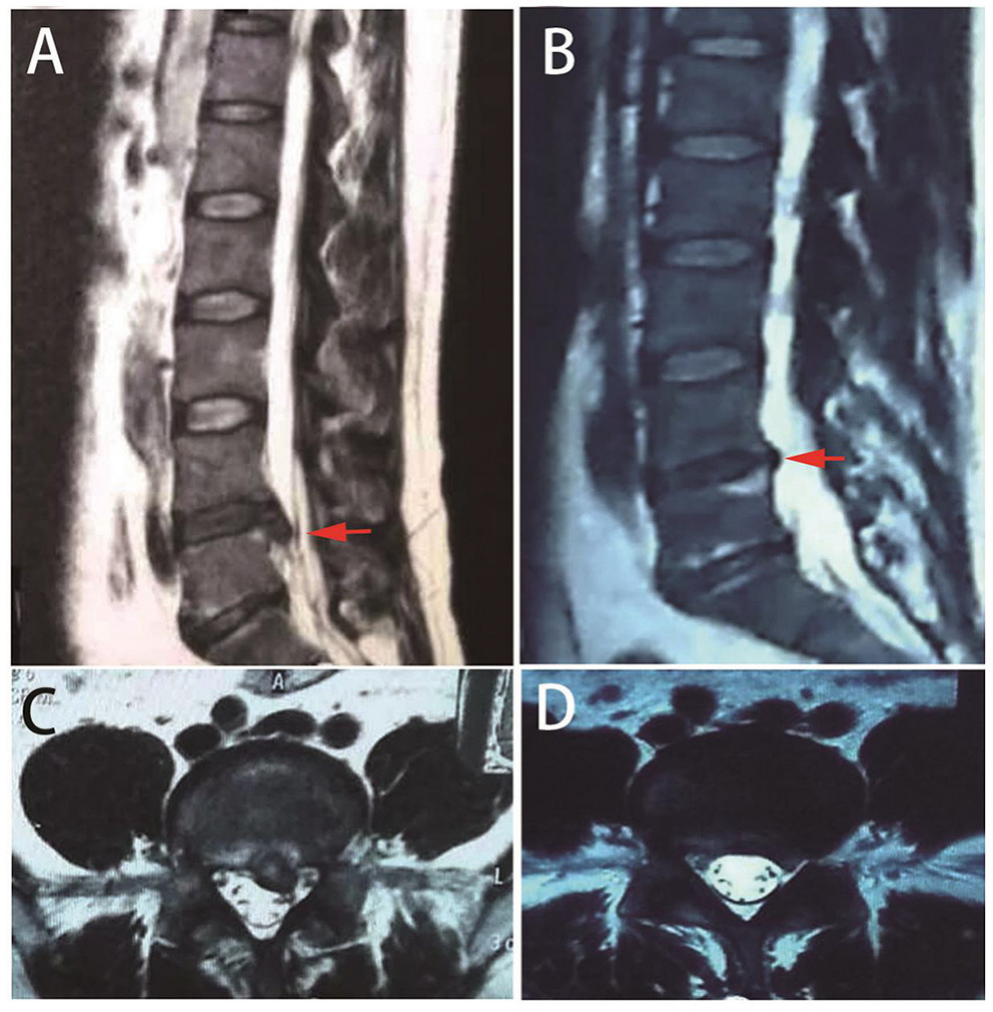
Repeated MRIs of a typical case with spontaneous resolution. A 39-year-old male patient presented with low back pain and sciatica. MRI indicates lumbar disc herniation at L5/S1 [(A,C) red arrows]. One year later, repeated MRI indicates herniation resolution [(B,D) red arrows].
